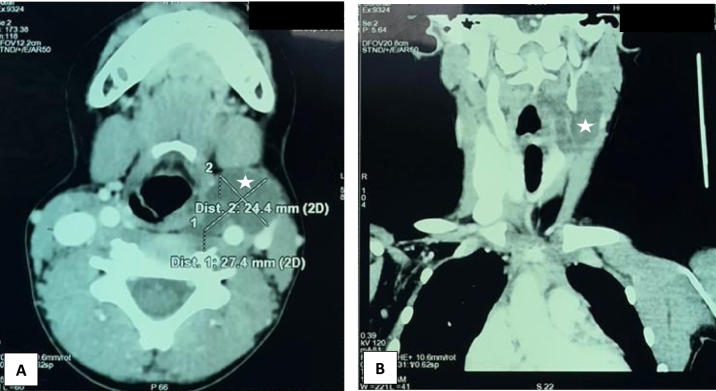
Cervical CT scan on axial (A) and coronal (B) images: Left latero-cervical multiloculated cystic mass (white star), measuring 27 ∗ 24 mm, with a mass effect on the oropharynx.
